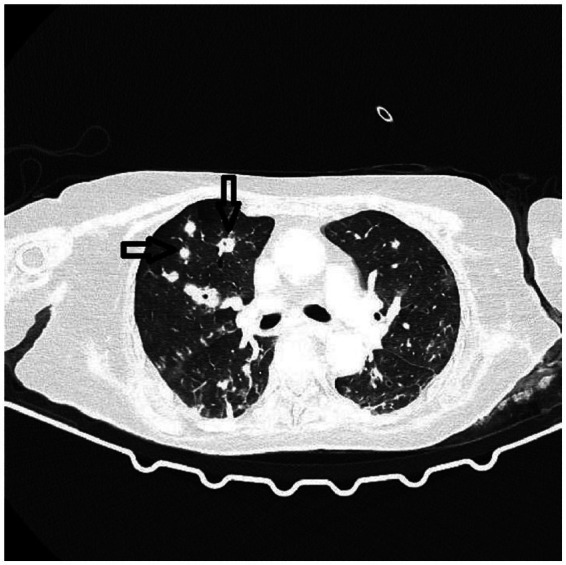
The CT scan shows dense, well-circumscribed lesions without a halo sign.
